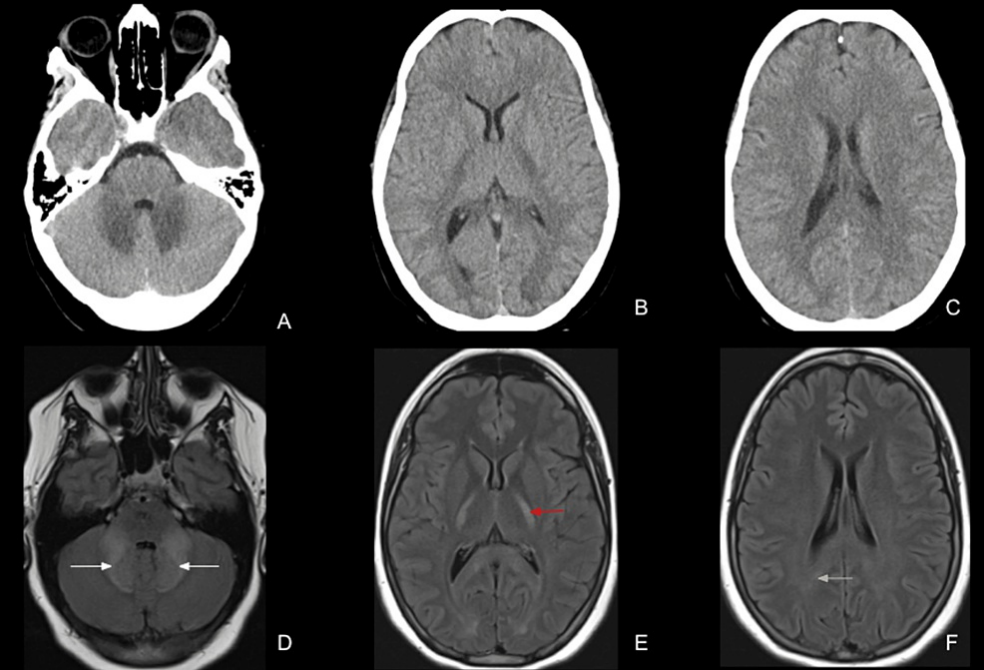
Top row: Axial CT scan of the head without contrast at the level of the cerebellum (A), internal capsule (B), and lateral ventricles (C), respectively. Bottom row: Accompanying axial FLAIR MRI at the level of the cerebellum (D), internal capsule (E), and lateral ventricles (F). Symmetric abnormal bright signals (indicated by arrows) are noted in the cerebellar peduncles (D) (white arrows), posterior limb of the internal capsule (E) (red arrow), and the occipital periventricular white matter (F) (yellow arrow).
